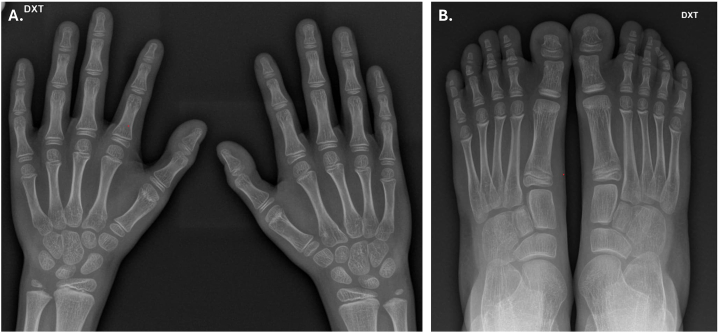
X-ray imaging of the hands (A.) and feet (B.).
